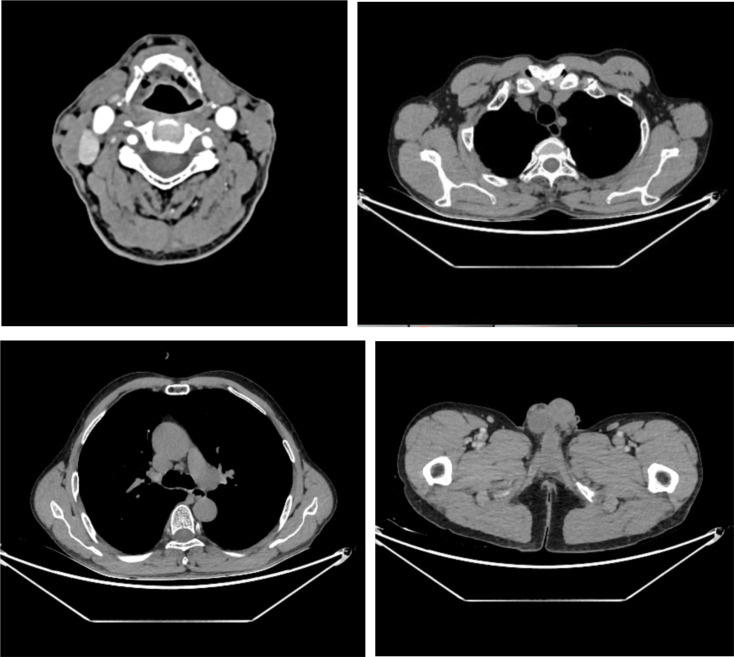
CT scan (July 15, 2025) showing normal-sized lymph nodes in the bilateral cervical, axillary, supra- and infraclavicular fossae, mediastinum, abdominal cavity, retroperitoneum, and bilateral inguinal regions.
